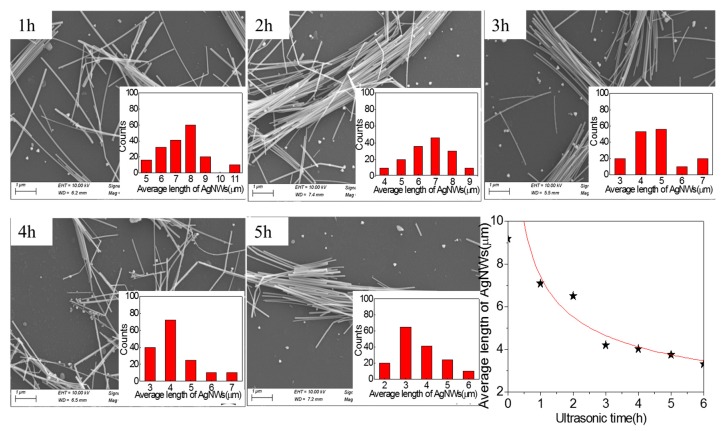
SEM images of AgNWs (1.67 mg·mL^−1^) treated with ultrasonic power 100 W and different ultrasonic time and the relationship of average length of AgNWs with ultrasonic time.
